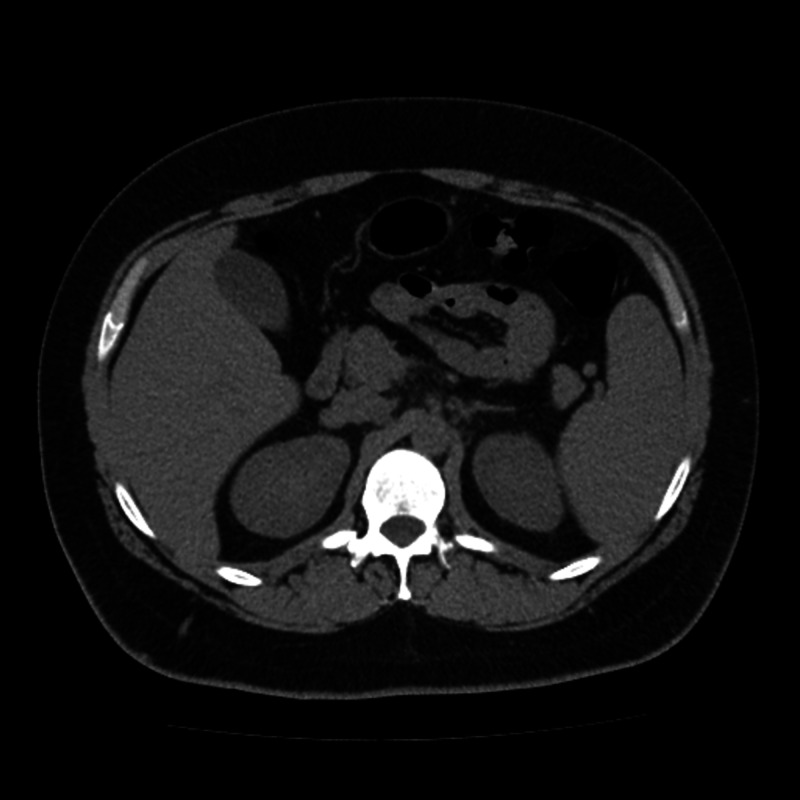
Normal abdominal CT of the patient CT: computed tomography
